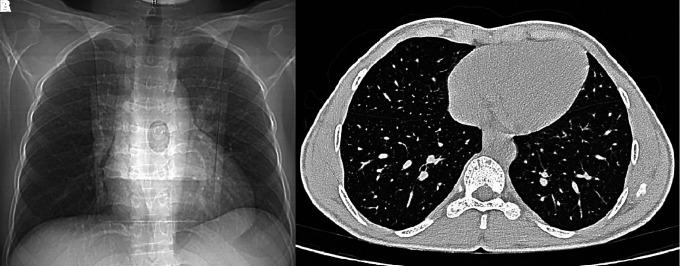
X-ray chest posteroanterior view (A) showing mildly prominent bronchovascular markings and crowding of ribs and HRCT thorax (B) axial plane showing normal lung parenchyma and mild flattening of anterior chest wall. The restrictive pattern on spirometry and DLCO when corroborated with chest imaging indicate the cause of restriction to be extraparenchymal, due to chest wall deformity in diabetic cheiroarthropathy.
